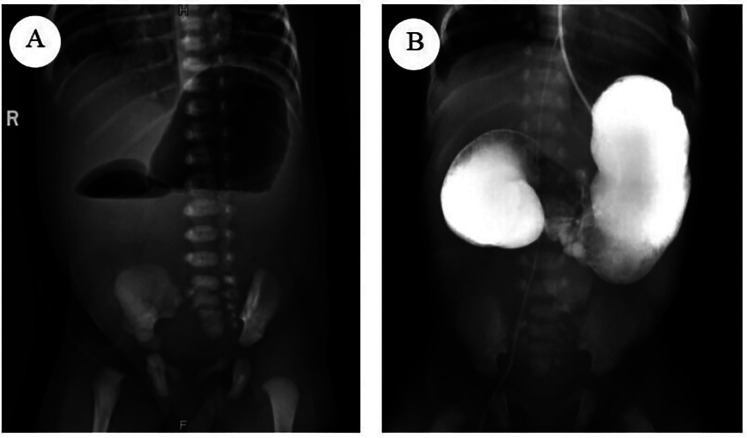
(A) The upright abdominal radiograph reveals the characteristic double-bubble sign, indicative of duodenal obstruction. (B) The Upper gastrointestinal contrast study revealed an enlarged gastric lumen, with minimal contrast medium entering the duodenum and small intestine.
